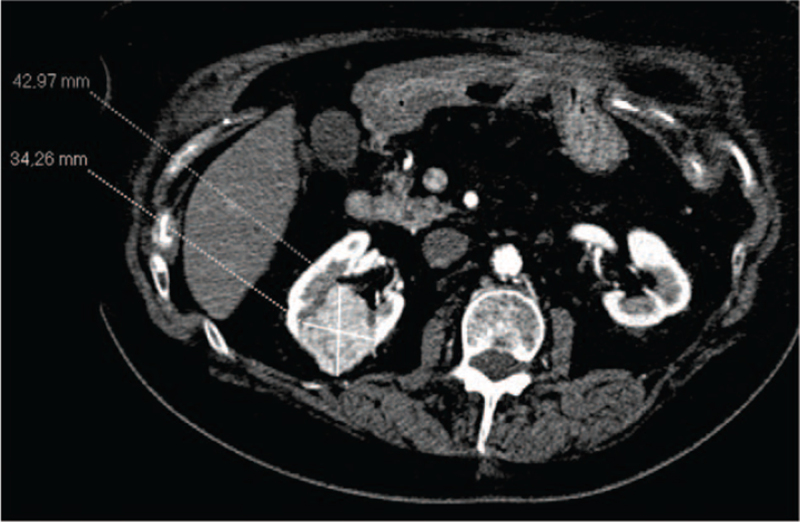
Abdomen CT scan showing the measurement of the hypervascular tumor lesion in the right kidney.
